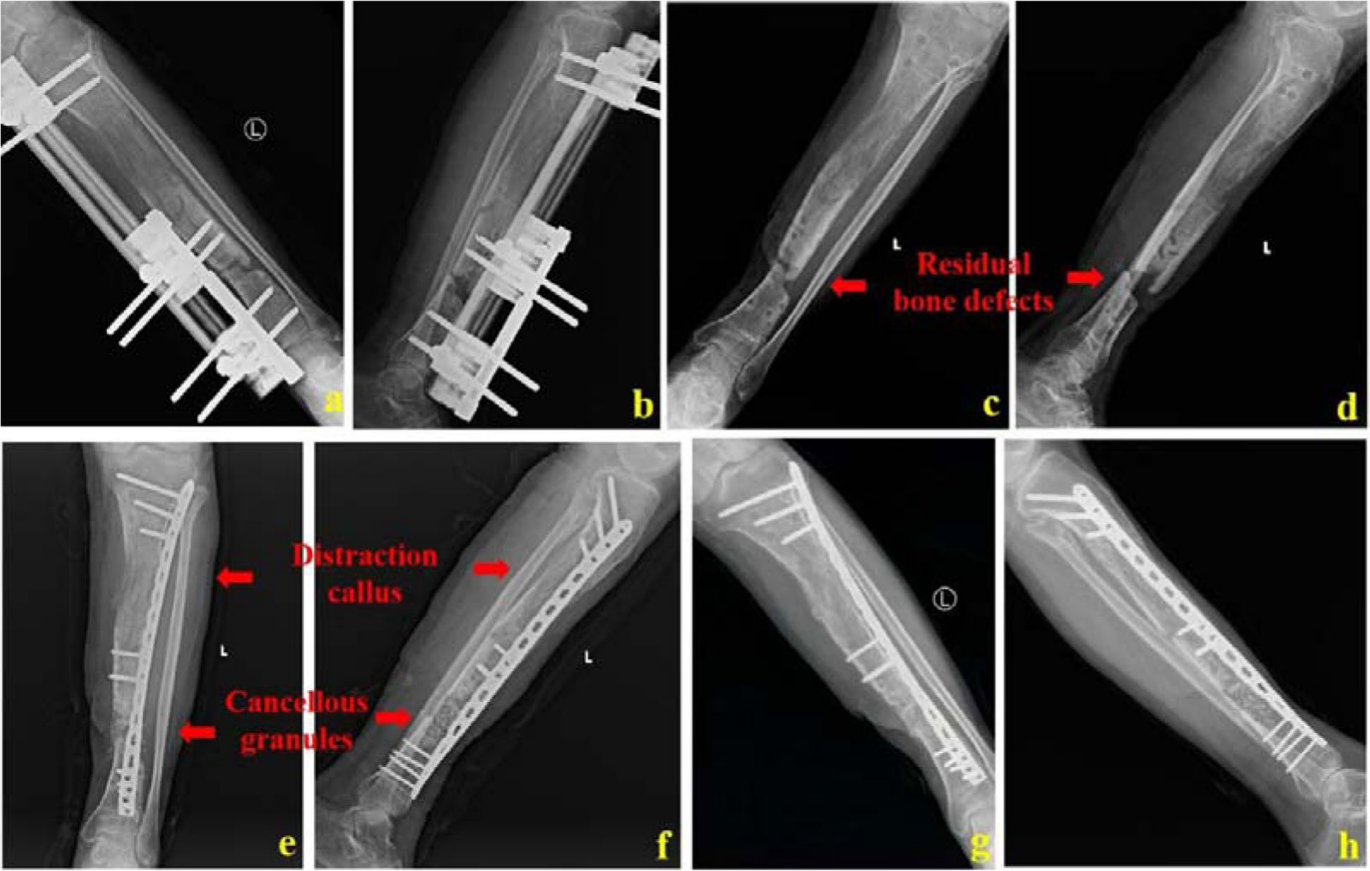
The 55-year-old male was treated by bone transport combined with bone graft and internal fixation technique. a and b: The bone defect length was shortened to 3.5 cm when the single-arm external frame was ready to be removed; c and d: X-ray images after removing the transport frame; e and f: X-ray images after cancellous bone graft and plate fixation; g and h: One year after bone graft, the bone defect healed well
